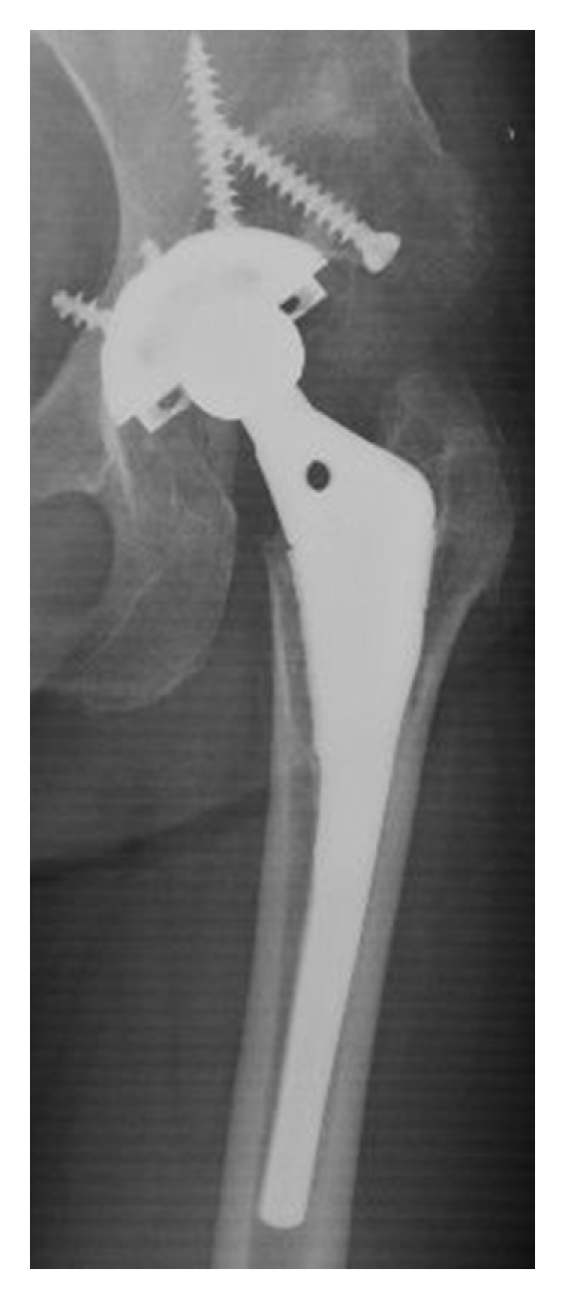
The AP radiograph of the left hip at 15 years after THA. The biological fixation of the stem was bone ingrown fixation.
